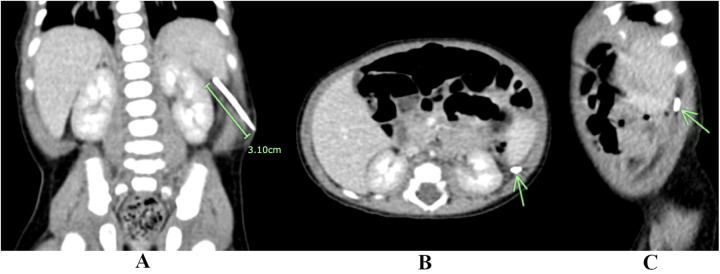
Computed tomography of the abdomen and pelvis. (A) Coronal view confirming a pin-like foreign body at the left flank measuring 3.1 cm (green line), with its blunt end extending to the splenorenal fossa. (B) Axial view demonstrating the blunt end of the foreign body (green arrow) in close proximity to the spleen, with associated reactive inflammatory changes in the subcutaneous tissues and minimal perisplenic fluid. (C) Sagittal view revealing the foreign body in cross-sectional profile (green arrow), confirming a lateral-to-medial trajectory of penetration through the left flank soft tissues.
